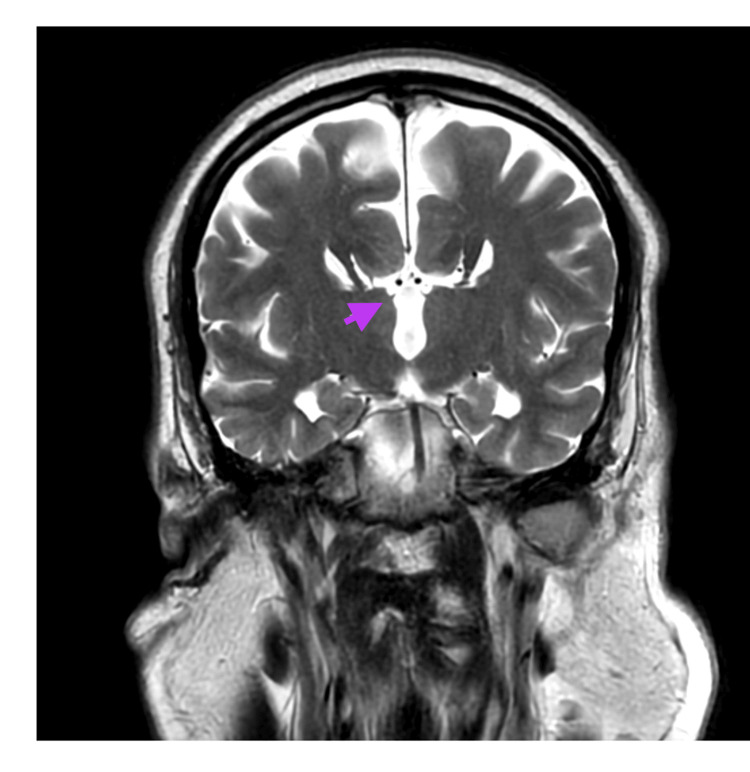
T2 propeller MRI brain coronal view displaying the “moose” sign characteristic of corpus callosum agenesis.
